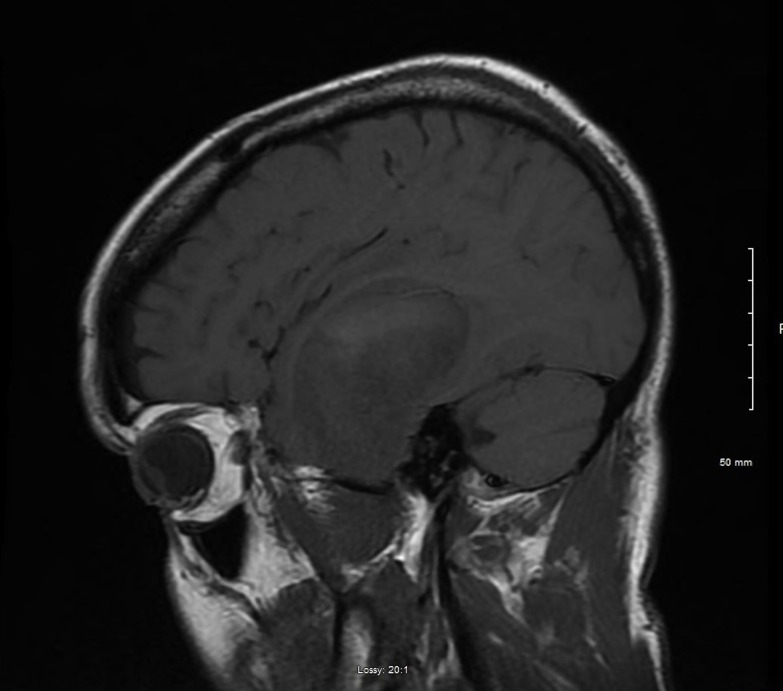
Brain MRI, Sagittal View Without Contrast Enhancement
